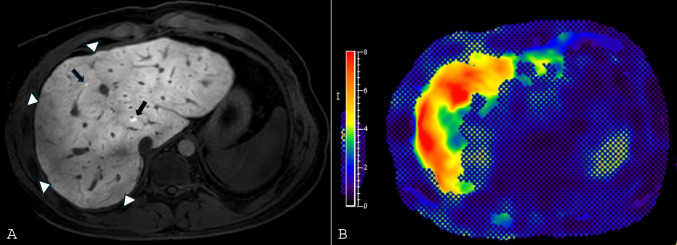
21-year-old female status post Fontan palliation with Fontan associated liver disease. A) Axial hepatobiliary phase MR image, obtained after administration of gadoxetate disodium, demonstrates a peripheral nodular contour of the liver with some areas of relative hypoenhancement of the liver parenchyma, predominantly in the liver periphery (arrowheads) representing areas of fibrosis. The left lobe of the liver is also mildly hypertrophied. Two small lesions that are retaining the hepatobiliary contrast agent are present and consistent with FNH-like lesions (*arrows*). B) Stiffness map from MR elastography reveals abnormally elevated stiffness of the liver parenchyma (indicated as higher stiffness parenchyma colored red per the scale on the left side of the image). This patient’s mean liver stiffness was 3.5 kPa, which is abnormally elevated
